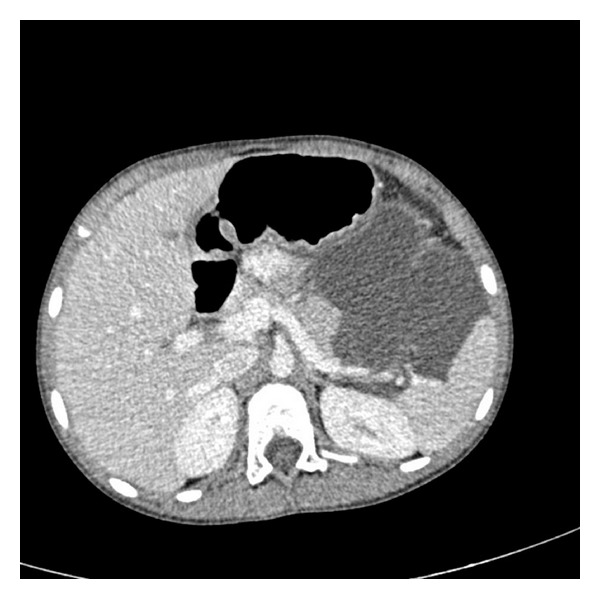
Contrast-enhanced CT axial scan shows retroperitoneal lobulated-septated cystic mass between spleen, stomach, and pancreas. Splenic vein and artery borders are in the cystic mass. Also cystic mass reaches to the pararenal space.
